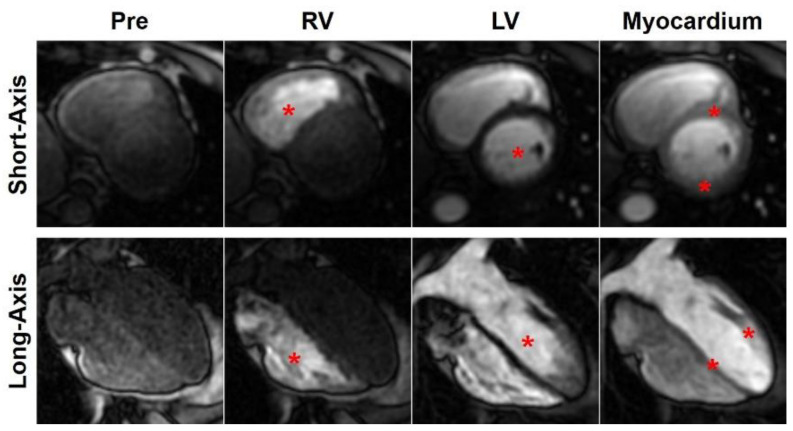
Myocardial perfusion imaging. Short-axis (top) and long-axis (bottom) series of T1-weighted saturation-recovery perfusion images acquired before contrast injection (pre), and at times of bolus arrival in the right-ventricular (RV) blood pool, left-ventricular (LV) blood pool, and myocardium, as denoted by the red asterisks. The images show normal perfusion without any defects.
